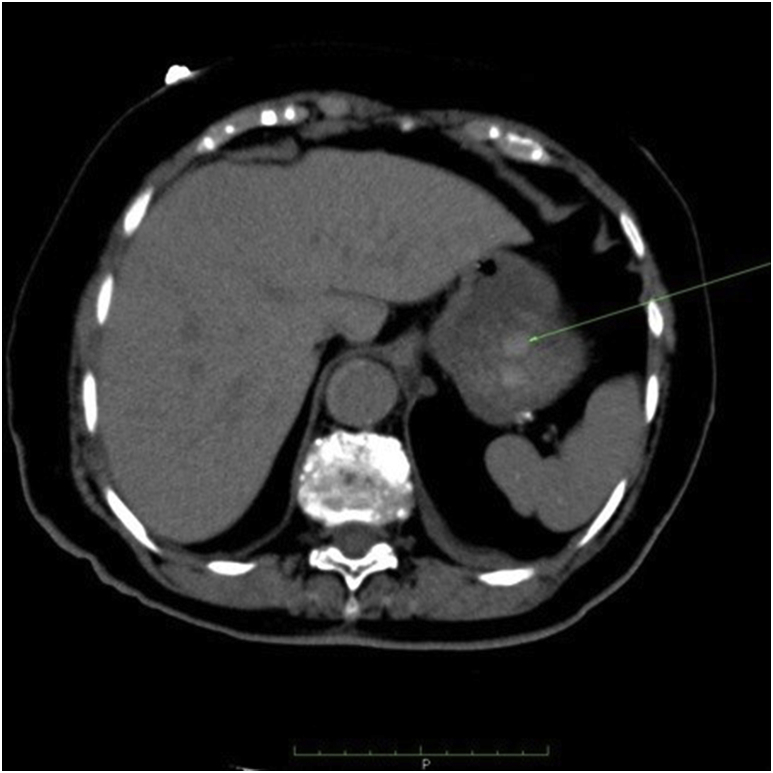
Gastric hematoma with a small amount of luminal blood on computed tomography.
